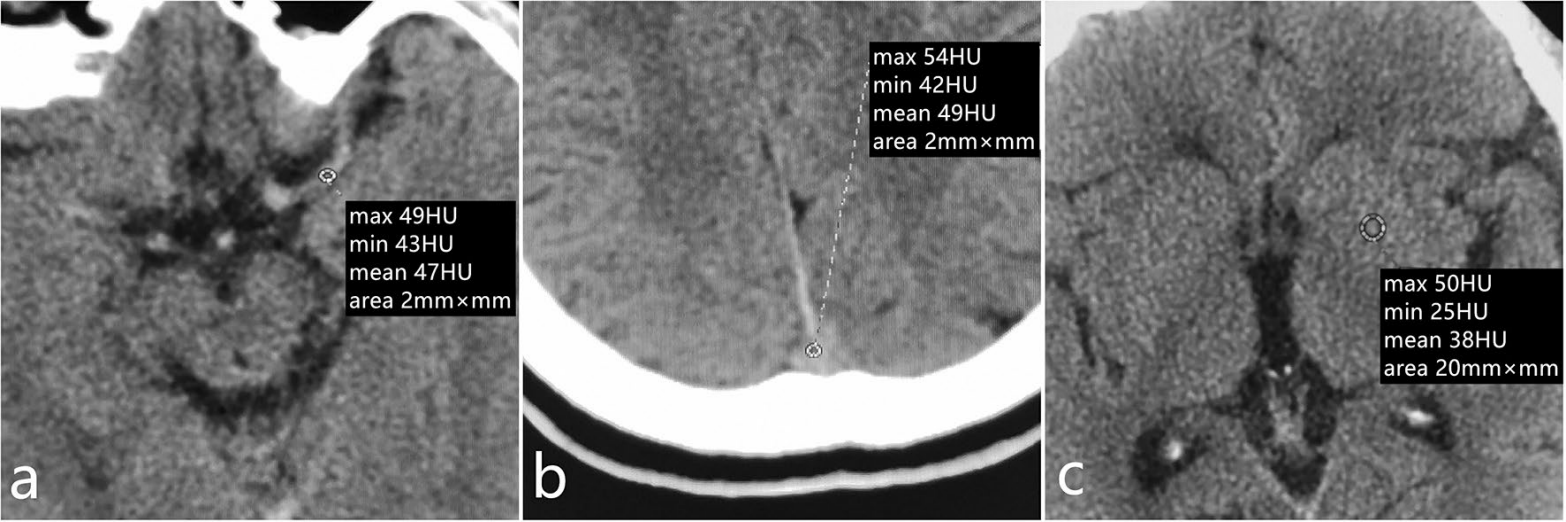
The CT values for basal ganglia, the middle cerebral artery, and the superior sagittal sinus were collected. The middle cerebral artery (a), the superior sagittal sinus (b), basal ganglia (c).
